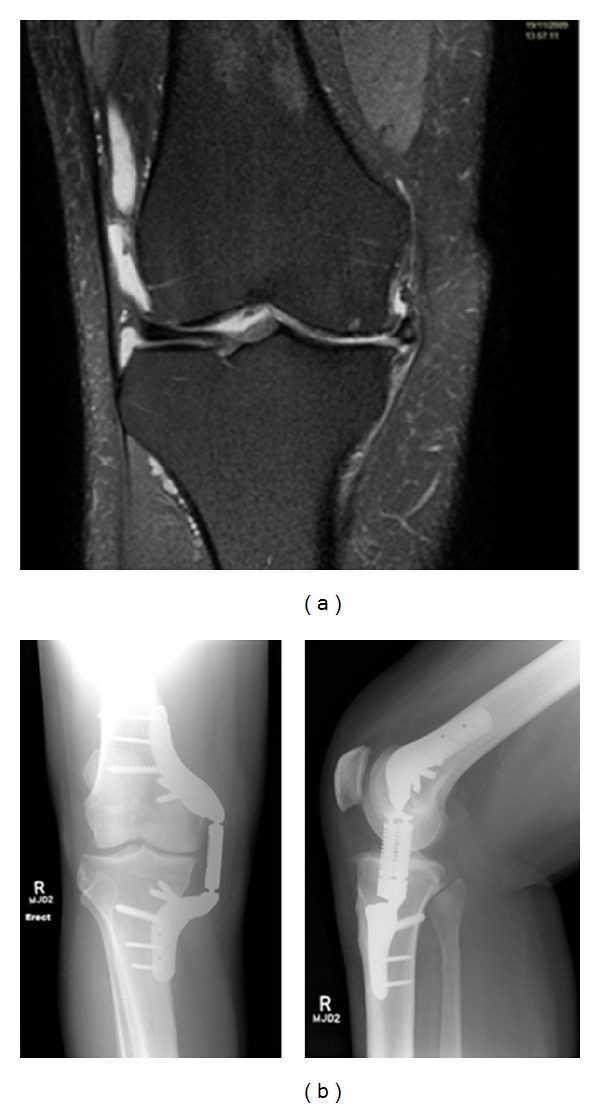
(a) Anterior view of the right knee with pre-treatment magnetic resonance imaging (a) and anterior (left) and medial (right) 1-year radiographs demonstrating the KineSpring System *in situ* (b).
